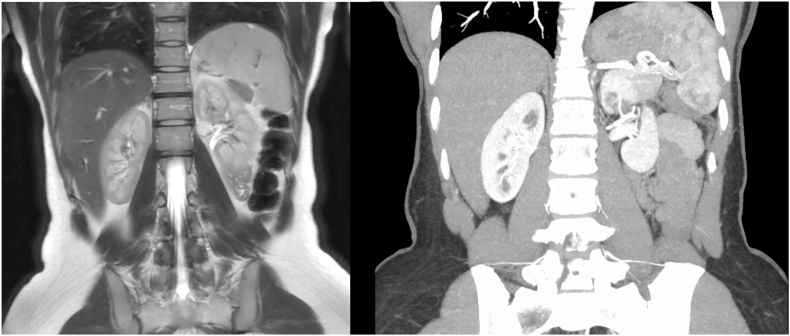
Preoperative imaging studies including MRI showing the mass found in the left kidney, which was measured to be 3.7cm (Left), and CT angiogram of accessory branching arteries to the tumor (Right).
